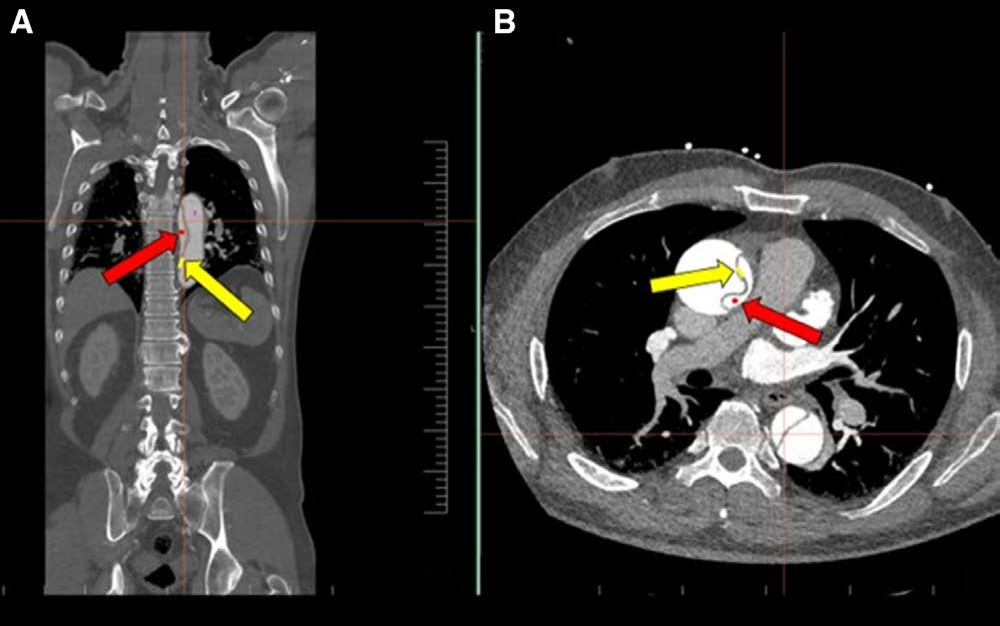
MIP reconstruction of CT angiography in the coronal (A) and transverse plane (B). Stanford type A aortic dissection is demonstrated, with a thin intimal flap (yellow arrow) visible within a fusiformly dilated ascending aorta. A slit-like, compressed true lumen (red arrow) is seen in the ascending aorta (B) and throughout the markedly dilated descending thoracic aorta (A). CT = computed tomography, MIP = maximum intensity projection.
